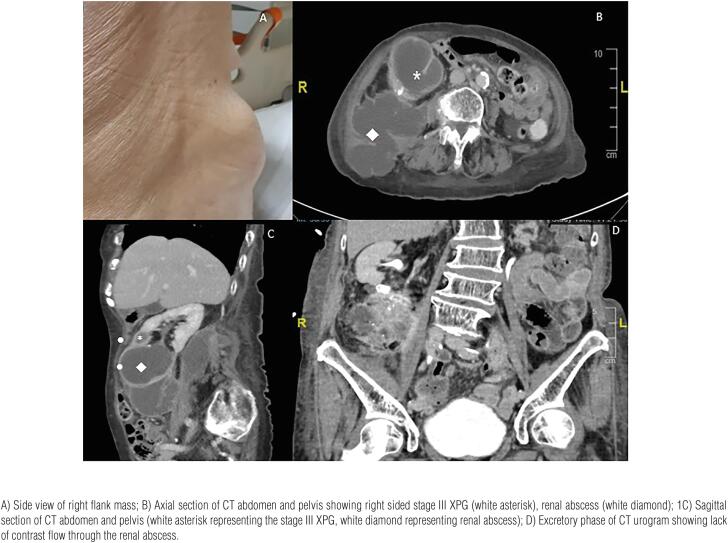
An 89 year-old female presenting with functional decline and a fall was discovered to have a right flank mass. She underwent CT abdomen and pelvis to evaluate the flank mass.
